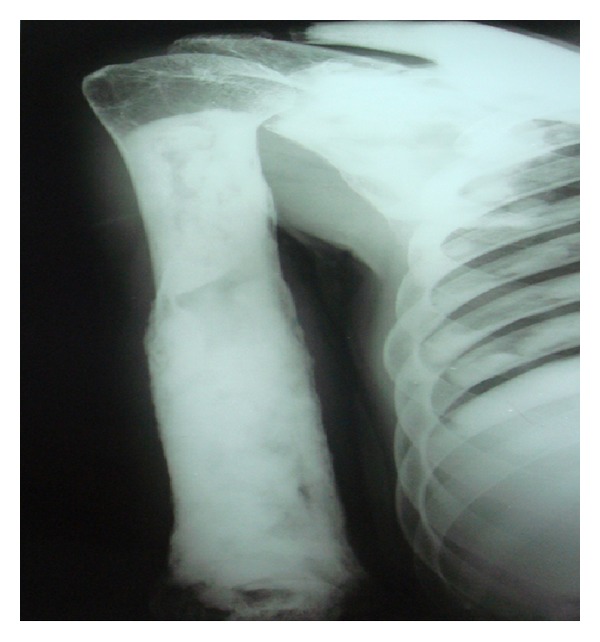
Radiograph of the humerus showing osteosclerosis of the humerus and scapula and typical “funnel-like appearance” (Erlenmeyer-flask deformity) in the humerus.
